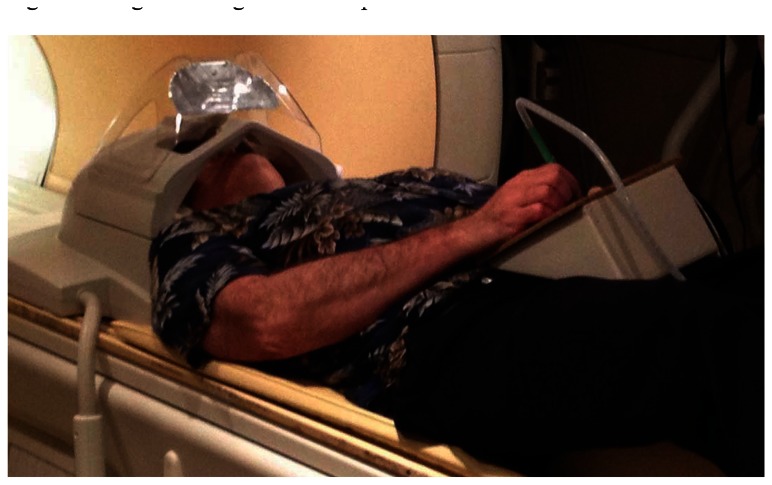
Tablet in use near the bore of the MRI scanner. The pen is connected to a fiber optic cable that goes through a waveguide that is positioned in the wall of the scanner room.
